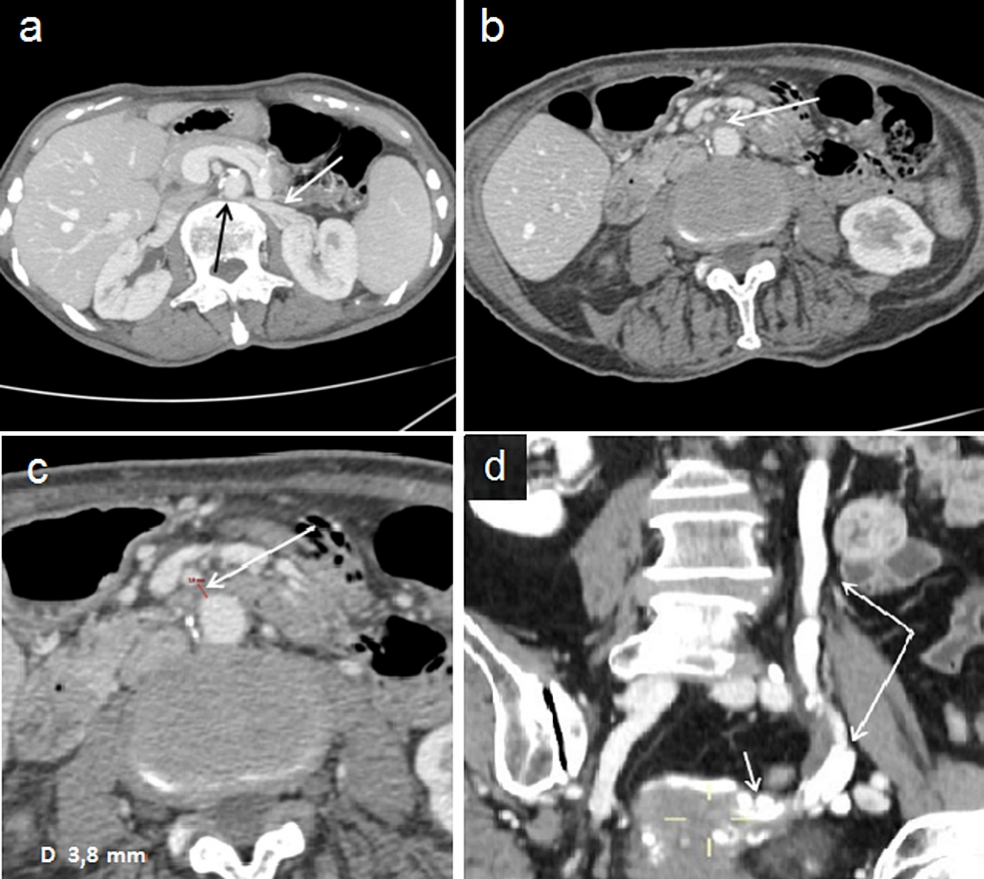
CT shows retroaortic LRV compression, duodenum compression, and left pelvic varicosities (a) A single 60-second post-contrast axial view shows LRV compression (black arrow) in aorto-vertebral space: LRV (with arrow). (b) A single 60-second post-contrast axial view shows duodenum (arrow) compression in aorto-mesenteric space. (c) In a 45-second post-contrast axial view aorto-mesenteric distance (arrow) appears reduced (6 mm). (d) A single 60-second post-contrast coronal view shows dilation of the left gonadal vein (long arrows) and ipsilateral gonadal plexus (short arrow).
